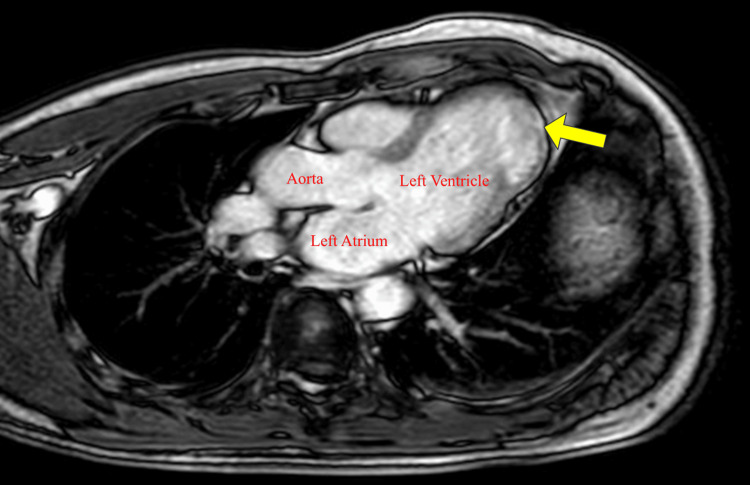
Magnetic resonance imaging (MRI) with late gadolinium enhancement (LGE) Contrast enhancement is observed in the sac of the pseudoaneurysm (yellow arrow).
